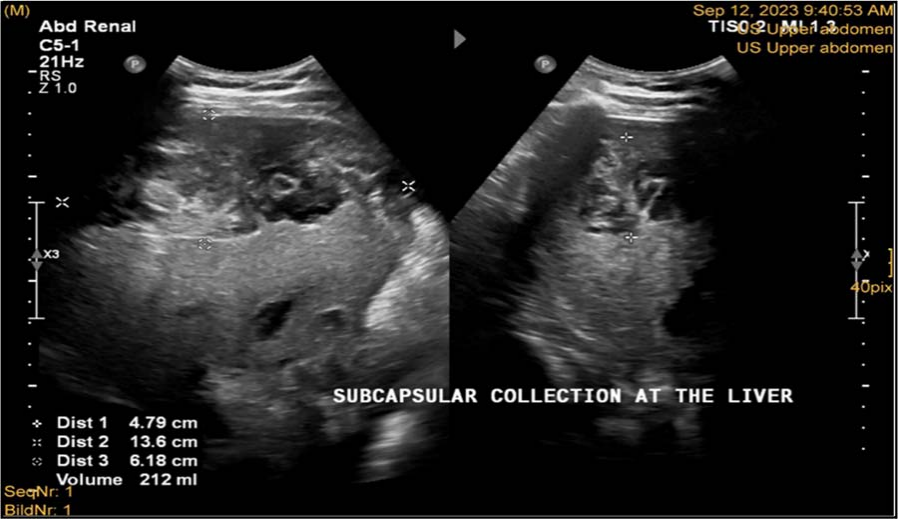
Ultrasonography postlab chole, shows right liver lobe subcapsular heterogenous density collection with liver surface being scalloped and pushed away from the capsule. Volume was about 212 ml. Morison pouch and Lino renal pouch was free.
